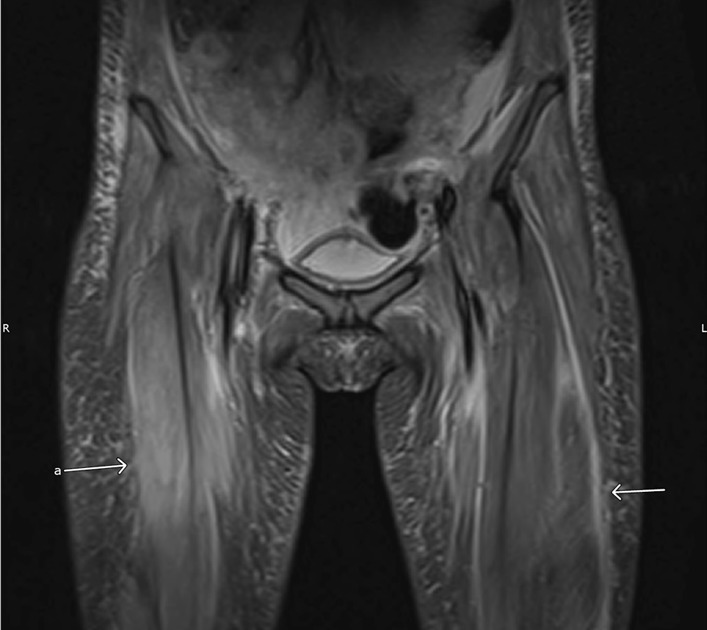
MRI Pelvis T1 fluid sensitive STIR sequence with arrows pointing to abnormal muscle signal of the muscles of quadriceps femoris indicating myoedematous changes
